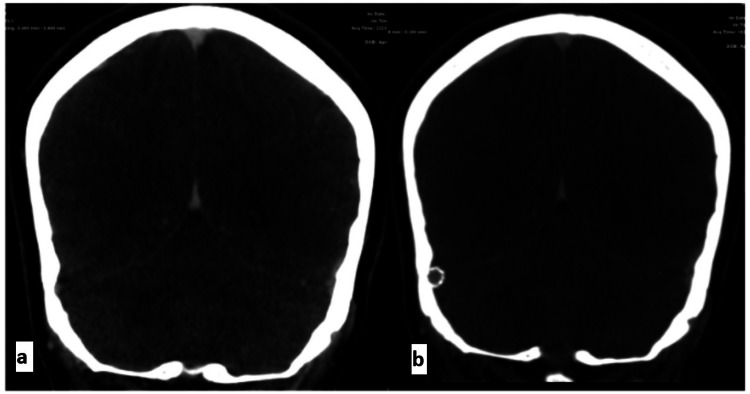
CTV before (a) and after (b) stenting showing good patency of the stent in the right transverse sinus CTV: computed tomographic venography
